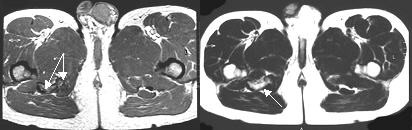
Pre-operative magnetic resonance imaging scans of the pelvis. The arrows point to the heterotopic bony masses.
